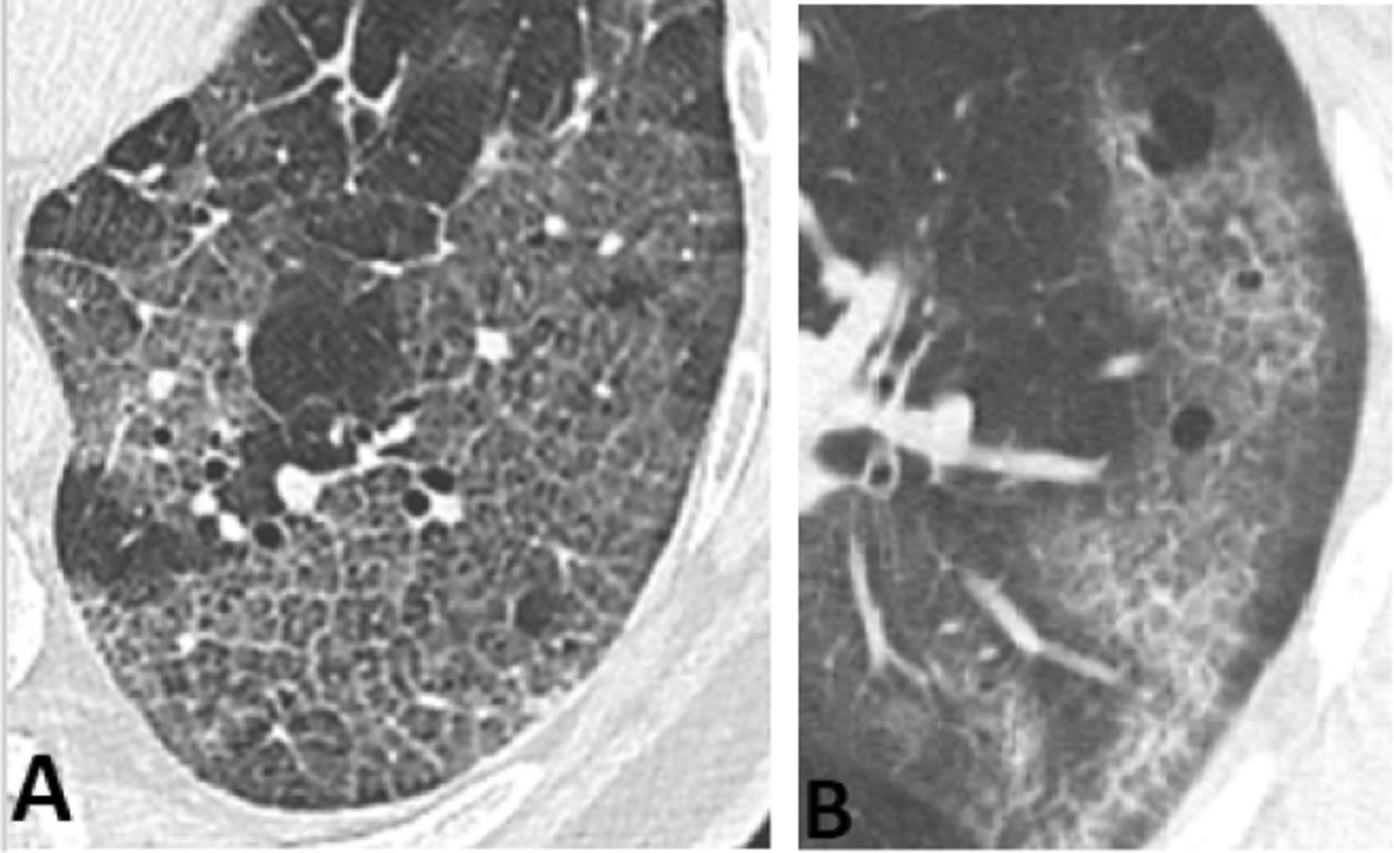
A a 70-year-old man without COPD/emphysema. B a 58-year-old man with COPD/emphysema. On axial CT images (region of interest) “crazy-paving” pattern. COPD—chronic obstructive pulmonary disease; CT—computed tomography
